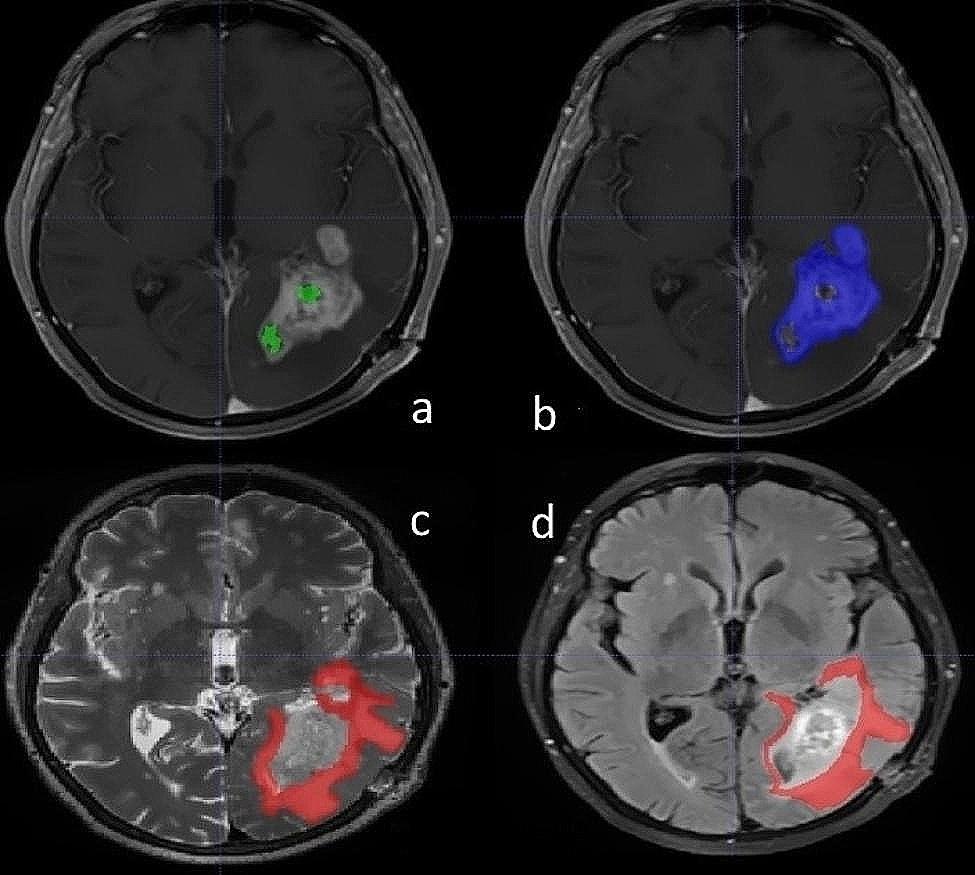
Tumor segmentation via ITK-SNAP by using input (a) T1W + GD image for tumor necrosis area, (b) T1W + GD image for enhancement area, (c) T2W image for edema area, and (d) T2W-FLAIR for edema area
